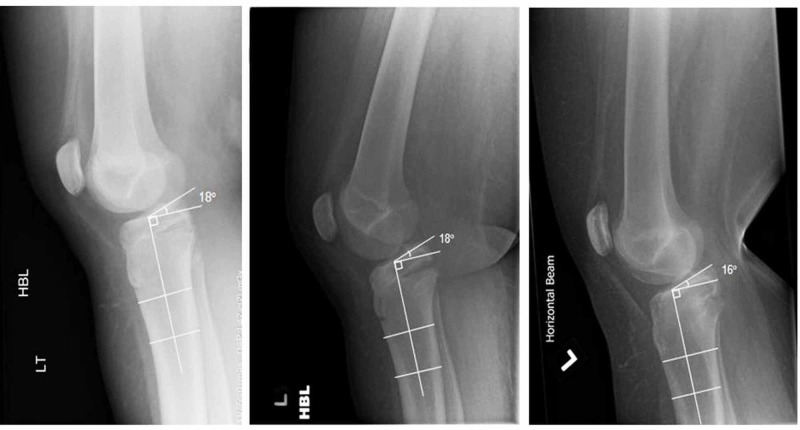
Lateral radiographs at (left to right) initial injury, four weeks post injury and 12 weeks post injury; tibial slope angle measurements displayed
